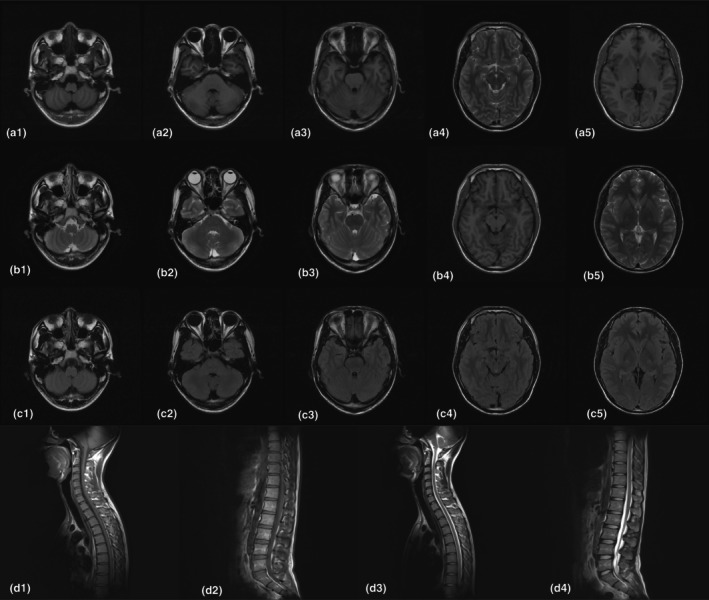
Magnetic resonance imaging (MRI). (a–c) Axial brain MRI scans showed no obvious abnormalities at five levels: cerebellum, pontocerebellar peduncle, brainstem, midbrain, and internal capsule. a = T1‐weighted MRI, b = T2‐weighted MRI, c = fluid‐attenuated inversion recovery. (d) Saggital spinal cord MRI scans showed no obvious abnormalities.
